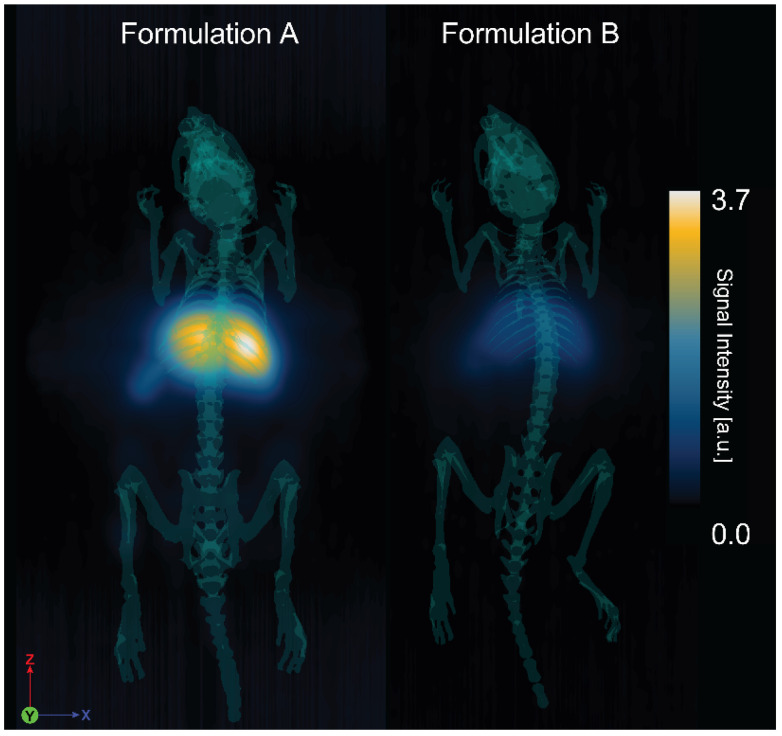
In vivo* imaging shows accumulation of particles from different formulations in liver and spleen. MPI 3D images overlayed with skeleton segments from CT image for anatomical referencing shows accumulation of the particles in the liver and spleen 24 hours after injection. For particles injected at the same dose, the difference in signal intensity is due to the performance of the formulation used.
